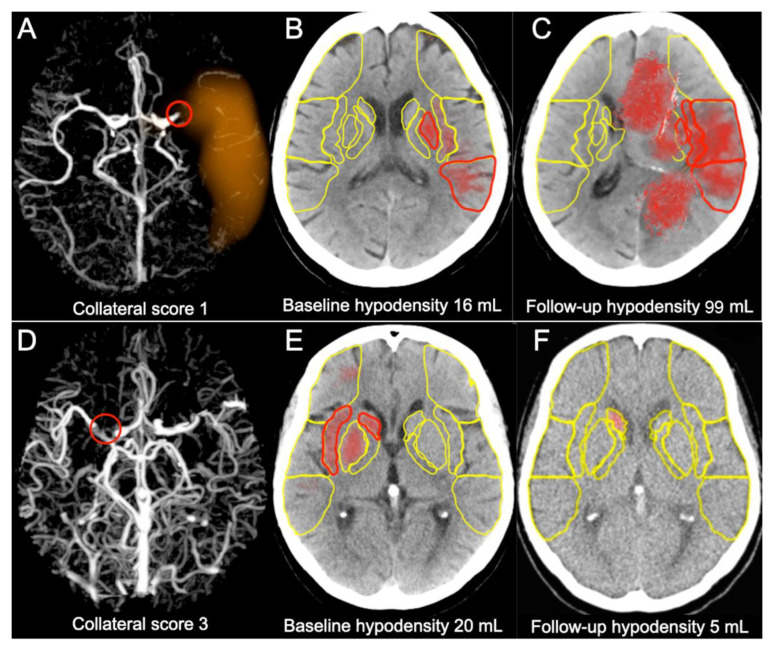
Representative cases with poor and good collaterals. Upper row presents the case of 79 y/o female treated 2 h and 43 min from onset of stroke with TICI 3. IVT was not administered due to the usage of oral anticoagulants: (A) left M1-MCA occlusion shown by red circle on timing-invariant CTA and poor collaterals scored as 1 by e-CTA; (B) e-ASPECTS assessed acute hypodensity volume for 16 mL on the baseline NCCT (red areas); (C) the follow-up NCCT revealed significant increase of hypodensity calculated for 99 mL by e-ASPECTS (red areas). Lower row presents the case of 72 y/o female treated 4 h and 50 min from onset of stroke with TICI 3: (D) right M1-MCA occlusion shown by red circle on timing-invariant CTA and good collaterals scored as three by e-CTA; (E) e-ASPECTS assessed acute hypodensity volume for 20 mL on the baseline NCCT (red areas); (F) the follow-up NCCT revealed significant decrease of hypodensity calculated for 5 mL by e-ASPECTS (red area). Regions used for calculation of ASPECTS are marked in yellow.
